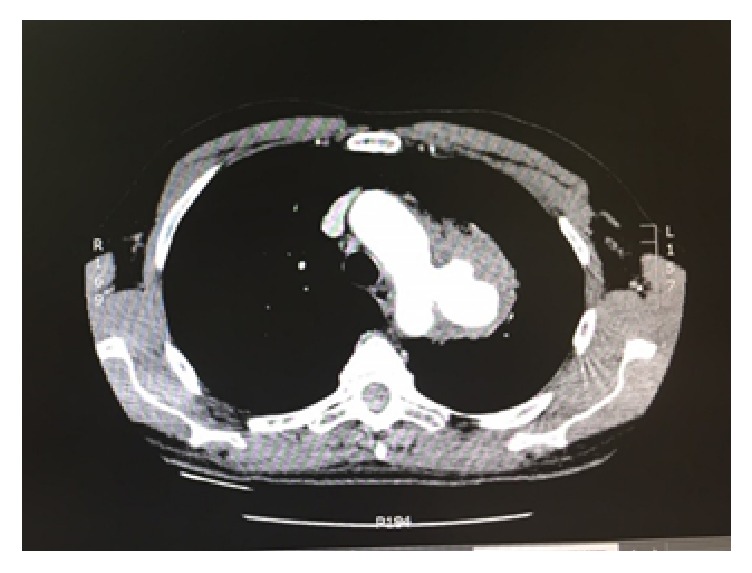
CT with intravenous contrast showed a 5,5 cm saccular aneurysm of descending thoracic aorta.
